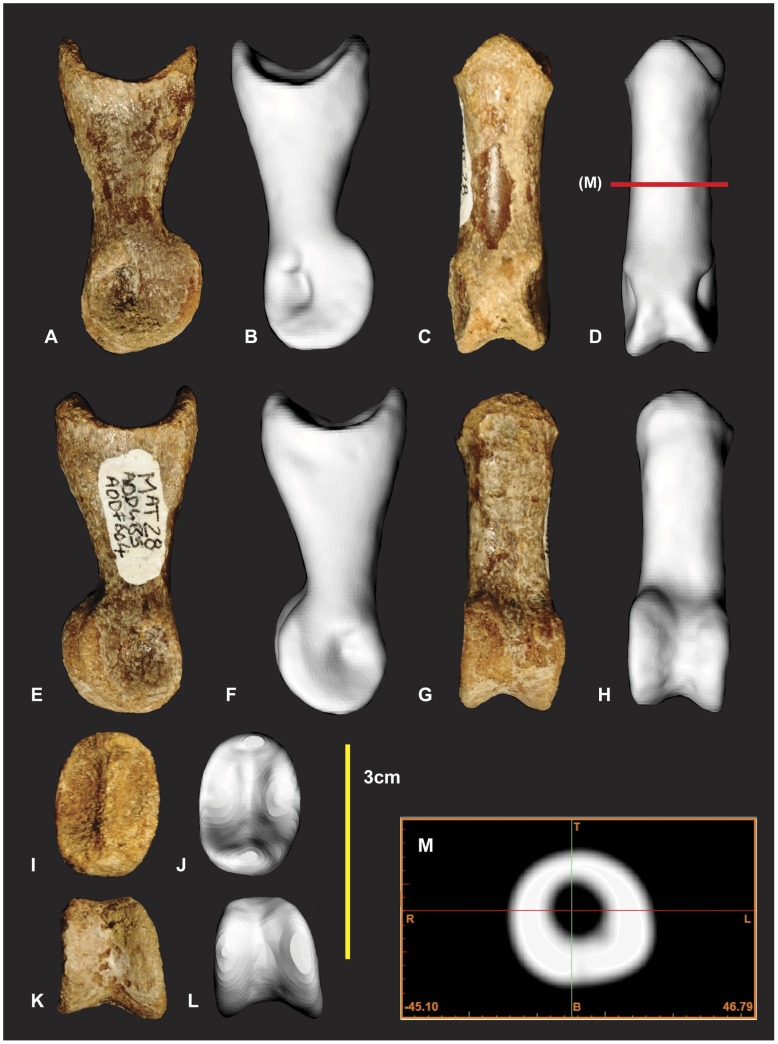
Right manual phalanx III-3. Right manual phalanx III-3 in medial (A & B), dorsal (C & D), lateral (E & F), ventral (G & H), mid shaft CT image (I).
